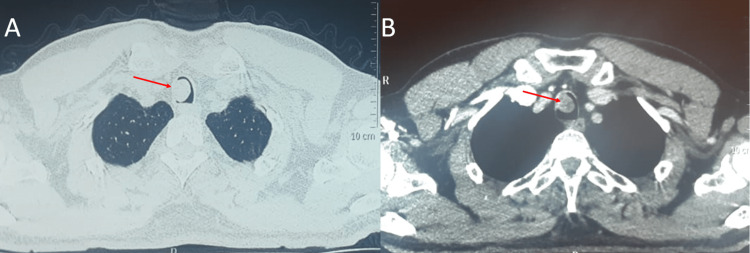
Thoracic CT scan, parenchymal (A) and mediastinal window (B), showing a round hypodense lesion within the trachea (red arrows).
